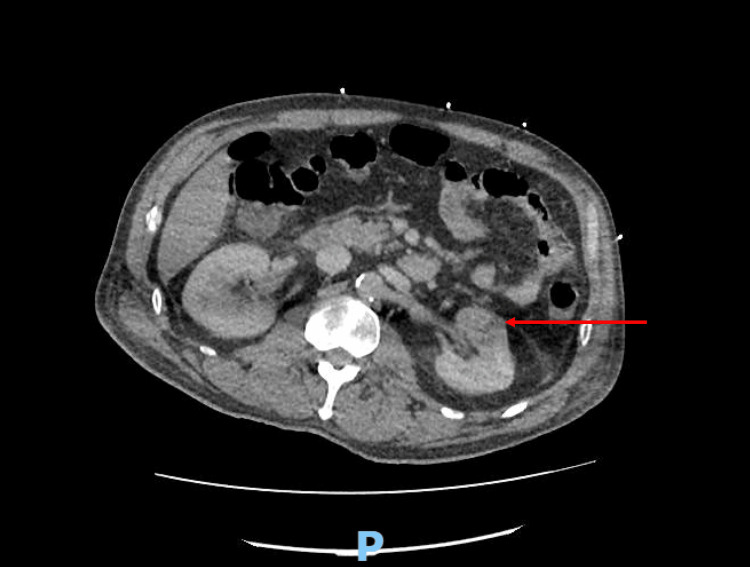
Abdominal computed tomography showing a low attenuation area in the left kidney indicating renal infarction.
